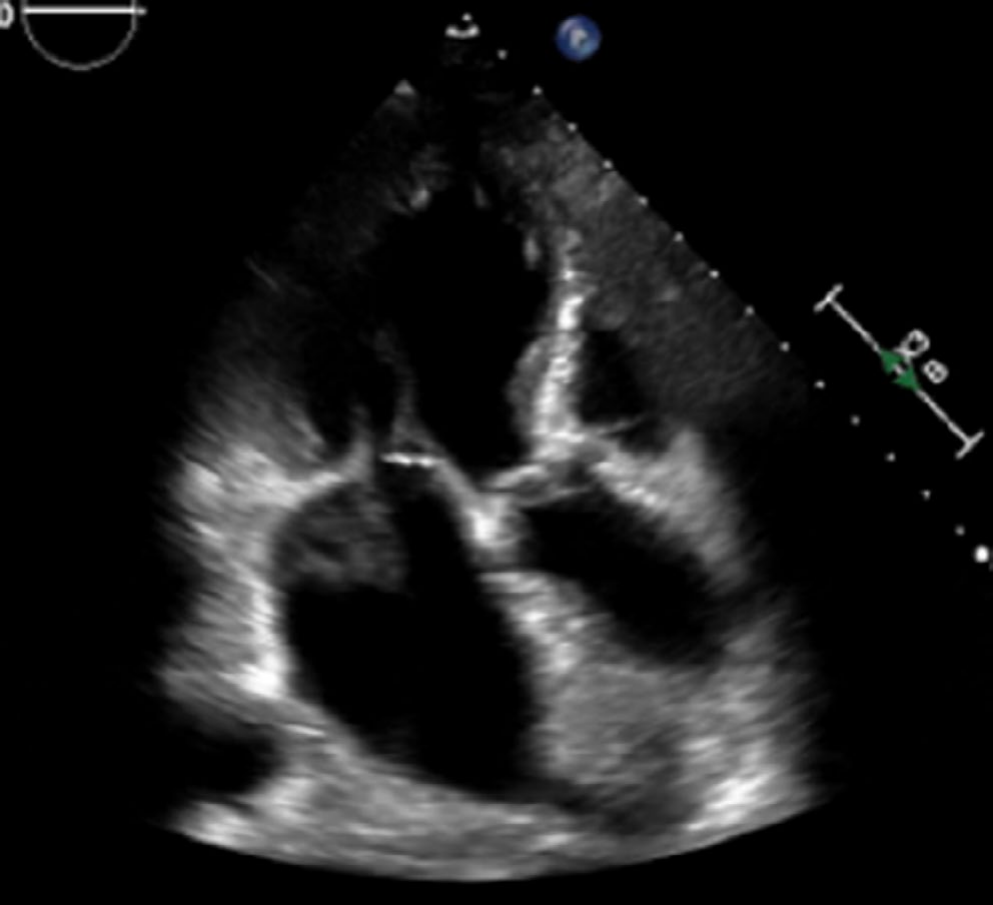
Transthoracic echocardiogram showing the improvement in hematoma postoperatively
